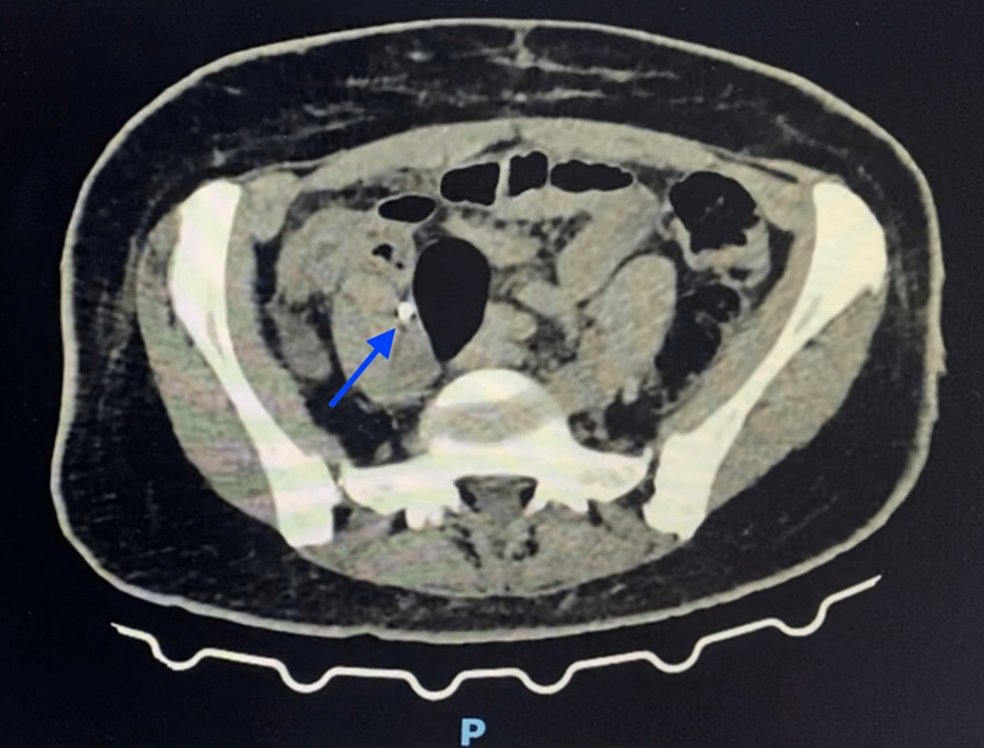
CT scan of the pelvis The image shows a moderate collection of liquid on the pelvis (marked by the blue arrow).
